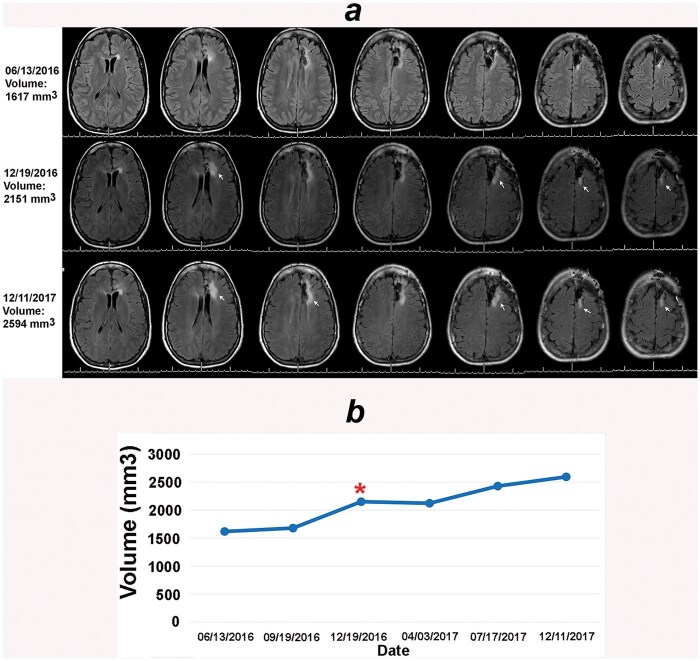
Volumetric analysis combined with the online change-of-point method detected progression on 19 December 2016 (see arrows) as compared to baseline on 13 June 2016. The columns in (A) represent sequential sections from the same MRI series. The MRI on 11 December 2017 was read as stable; arrows point to tumor growth as compared to the MRI of 19 December 2016. (B) The online change-of-point analysis detects progression at the red Asterix. The clinical notes considered all these MRIs as stable.
